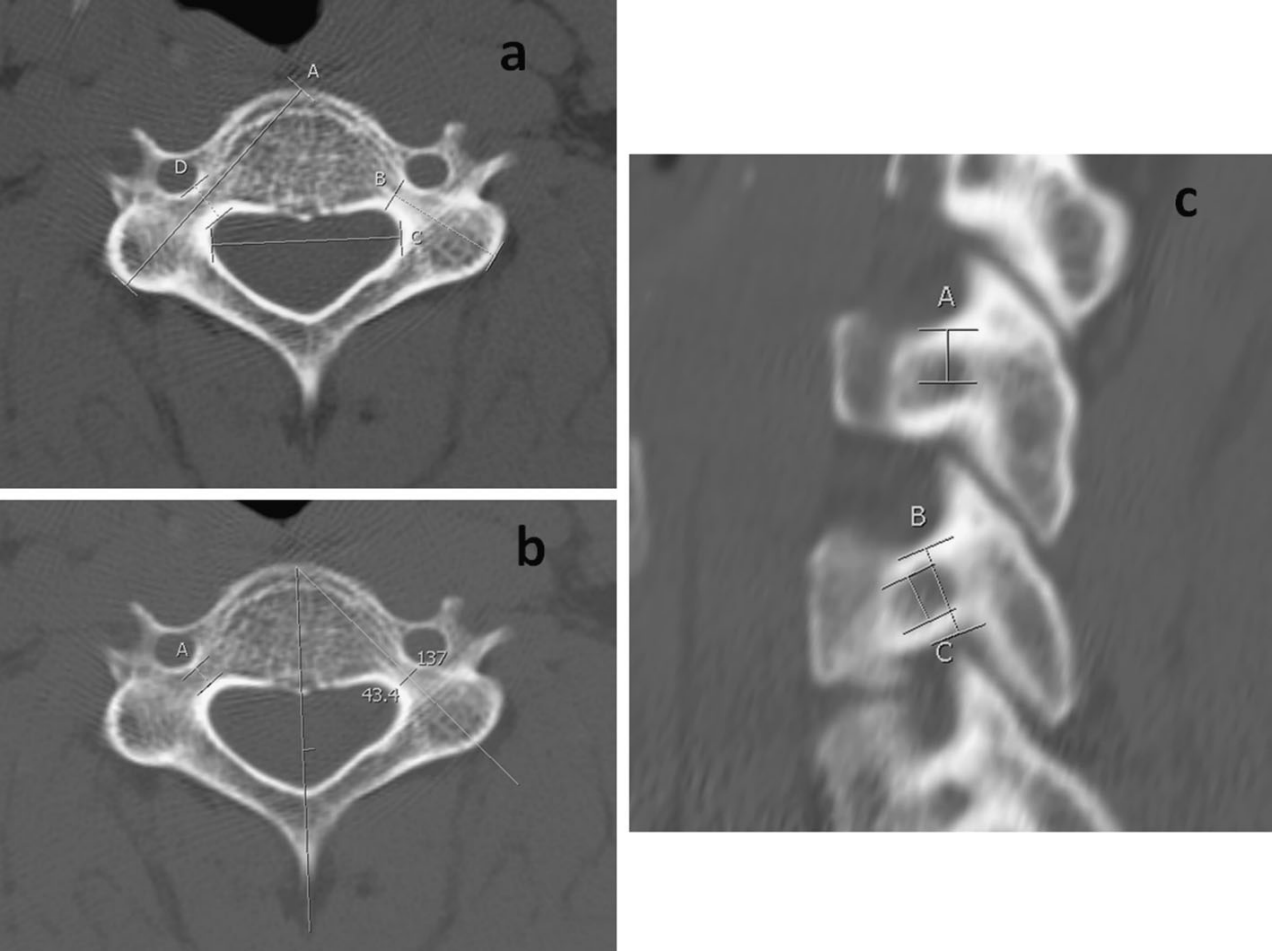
Morphometric measurements of the subaxial cervical spine pedicle. a Axial image of C4 vertebra on computed tomography scan showing the pedicle axis length (*A*), pedicle length (*B*), interpedicular distance (*C*) and outer pedicle width (*D*). b Axial image of C4 vertebra on computed tomography scan showing the inner pedicle width (*A*) and transverse angle, which in this particular case was 43.4°. c Sagittal reconstruction image of the cervical spine at the level of C3 and C4 showing the superior pedicle distance (*A*), outer pedicle height (*B*) and inner pedicle height (*C*)
